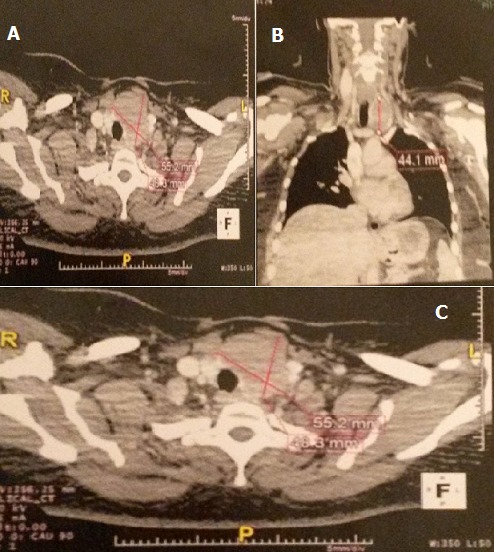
Computed tomography showed a thyroid nodule of the left lobe extended to the isthmus and the right lobe with anterior and posterior capsular rupture contracting close contact with the vascular axis left carotid-jugular plunging into the cervicothoracic away from the hole aortic arch (A,B,C)
